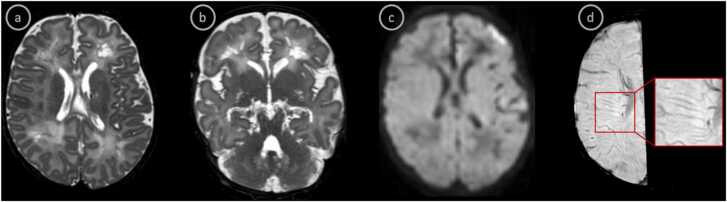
MRI-findings 28 days after symptom onset (aged 43^+1^ postmenstrual weeks). Panels (a) (T2 weighted - axial view) and (b) (T2 weighted - paracoronal view) show symmetrical periventricular white matter cystic infarction following the radiating pattern of draining medullary veins, which are seen on the susceptibility-weighted imaging (SWI) in panel (d). Note the lack of haemorrhage (no susceptibility artefacts on SWI - panel d) and lack of acute ischemic changes (no restricted diffusivity on diffusion weighted Imaging - panel c). Similar patterns of white matter injury may be observed in other RNA-viruses, such as Parechovirus and Rotavirus [4].
